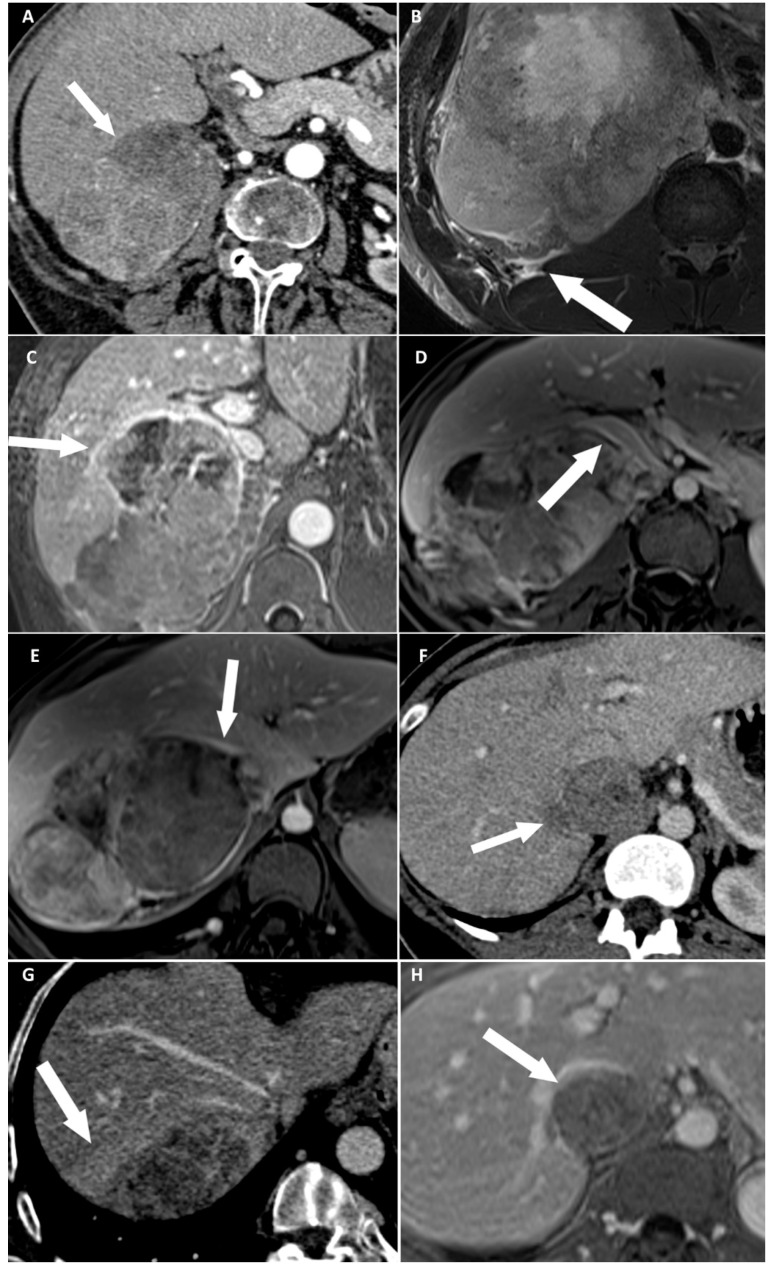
Categorical reading criteria. (A) A 76-year-old woman with a right-sided cortisol-secreting adrenocortical carcinoma (ACC) (Weiss score = 7, Ki67 = 13%), with direct liver involvement (DLI). CT image in the transverse plane obtained during the arterial phase following intravenous administration of iodinated contrast material shows disappearance of fat border between ACC and liver (arrow). (B) A 34-year-old man with a right-sided cortisol-secreting ACC (Weiss score = 9, Ki67 = 70%), with DLI. T2-weighted BLADE fat saturated (FS) image in the transverse plane shows periadrenal fat infiltration (white arrow). (C) A 57-year-old woman with a right-sided cortisol-secreting ACC (Weiss score = 9, Ki67 = 60%), associated with DLI. T1-weighted 3D volumetric interpolated breath-hold gradient-echo (VIBE) image in the transverse plane obtained during the venous phase following intravenous administration of gadoterate meglumine shows ACC contour disruption (arrow). (D) A 23-year-old woman with a right-sided cortisol-secreting ACC (Weiss score = 8, Ki67 = 50%), without DLI. T1-weighted VIBE image in the transverse plane obtained during the venous phase after intravenous administration of gadoterate meglumine shows macroscopic mass effect on inferior vena cava (arrow). (E) A 45-year-old woman with a right-sided ACC (Weiss score = 9, Ki67 = 16%), with DLI. T1-weighted 3D VIBE image in the transverse plane obtained during the venous phase after intravenous injection of gadoterate meglumine shows macroscopic mass effect on right hepatic vein (white arrow). (F) A 36-year-old woman with a right-sided noncortisol-secreting ACC (Weiss score = 7, Ki67 = 9%) associated with DLI. CT image in the transverse plane obtained during the venous phase following intravenous administration of iodinated contrast material shows focal ACC bulge (arrow). (G) A 55-year-old woman with a right-sided cortisol-secreting ACC (Weiss score = 6, Ki67 = 7 %), with DLI. CT image in the transverse plane obtained during the venous phase after injection of iodine based intravenous contrast agent shows periadrenal hepatic parenchyma enhancement (arrow). (H) A 36-year-old woman with a right-sided noncortisol-secreting ACC (Weiss score = 7, Ki67 = 9%) associated with DLI. T1-weighted VIBE image in the transverse plane obtained during the venous phase after intravenous administration of gadoterate meglumine shows ACC inclusion by hepatic parenchyma >180° (arrow).
